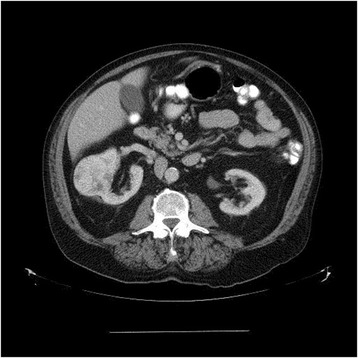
Contrast enhanced computed tomography of abdomen revealing solid enhancing mass arising laterally from right kidney
